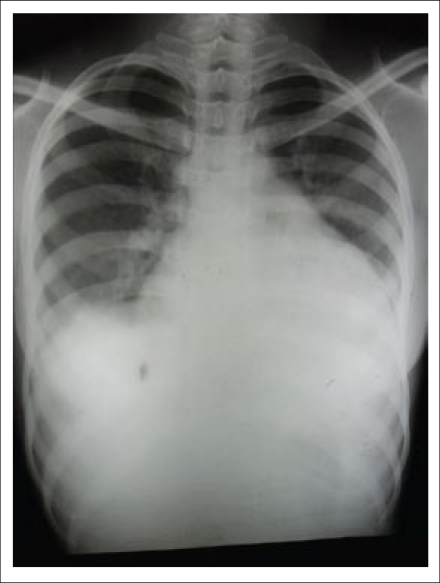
Chest x-ray showing enlarged cardiac shadow and bilateral pulmonary oedema
